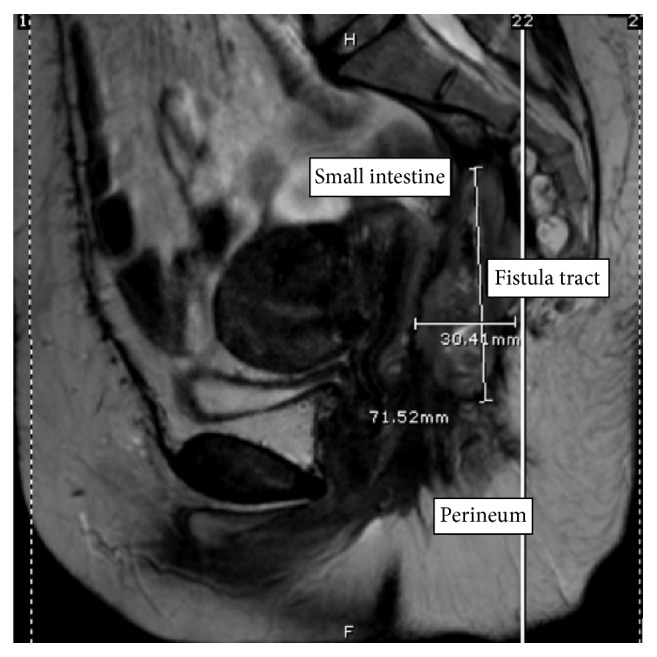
Sagittal view MRI of pelvis demonstrating 7 × 3 cm tract from perineum to presacral space.
